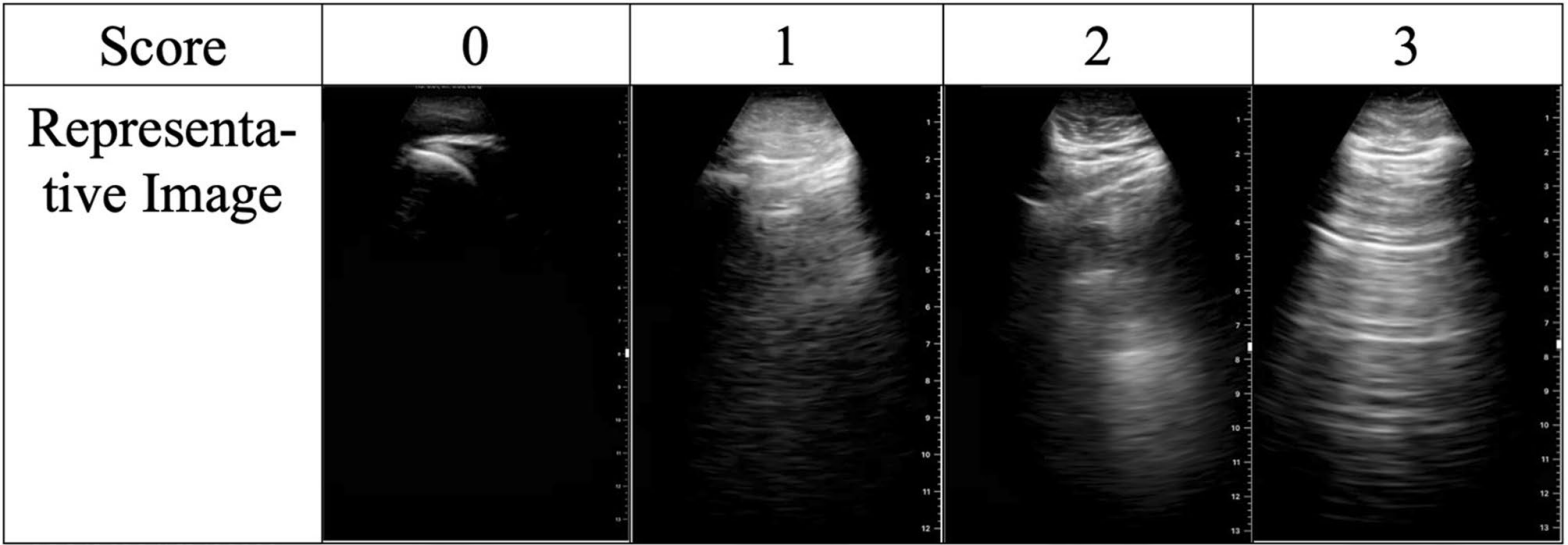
Scoring system used for grading novice- and expert-performed lung ultrasounds.
